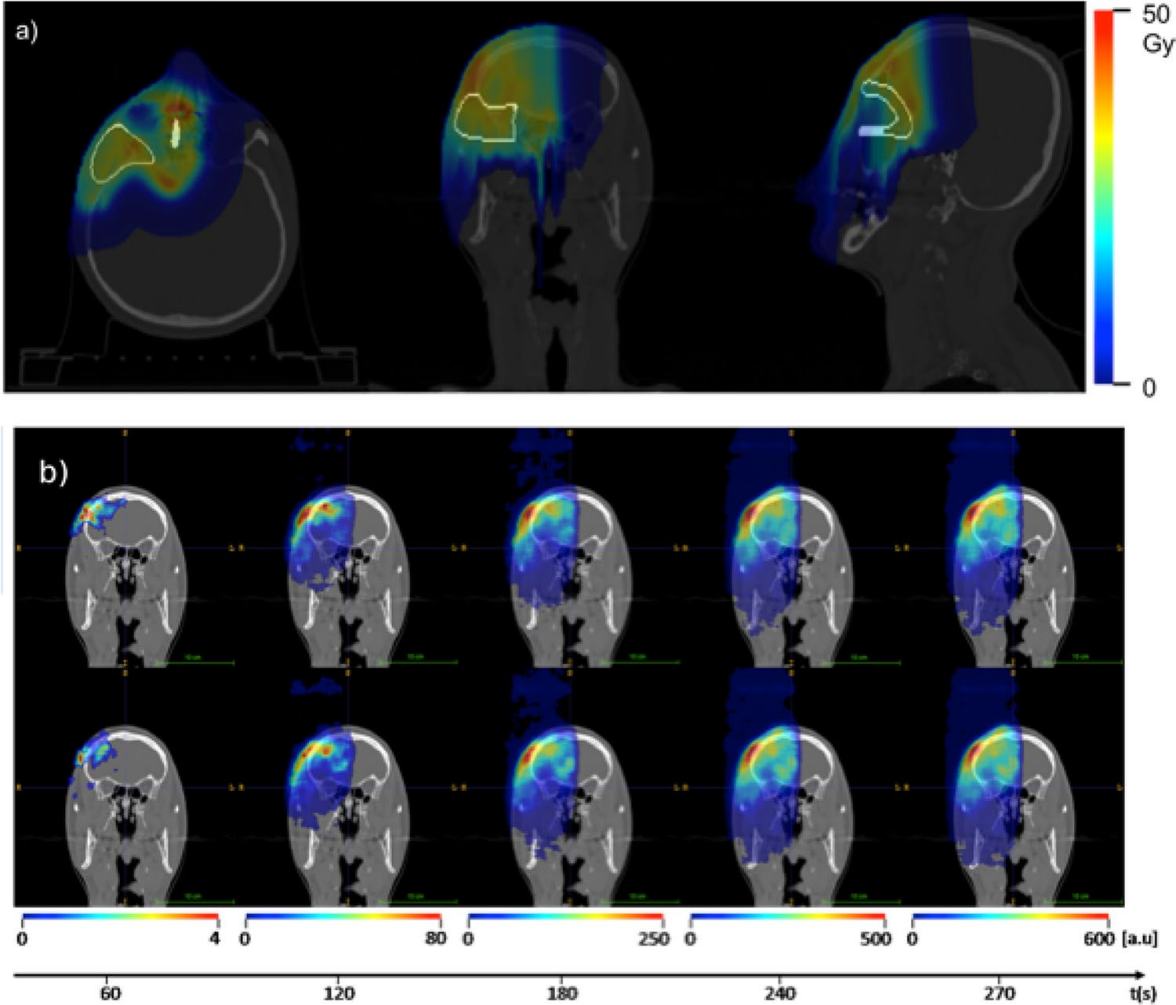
(a) Treatment plan and set up. Axial (left), coronal (centre) and sagittal (right) sections of the patient CT with the planned dose distribution to be delivered in the beam field monitored with the INSIDE in-beam PET system and the Clinical Target Volume (CTV) superimposed in white. (b) Time Evolution of a 2D slice of the detected beam-induced activity superimposed to the patient Computed Ttomography (CT) used for dose planning. The top and bottom rows refer to the first (December, 1st, 2016), and second (December, 2nd, 2016) acquisition days, respectively. The shown images correspond to 3D activity map reconstructions at the end of every minute, starting from the beginning of the treatment. An additional image corresponding to the whole treatment plus 30 s after-treatment is also shown. The image look-up tables refer to different intensity scales because of the different amounts of data integrated during the time intervals.
